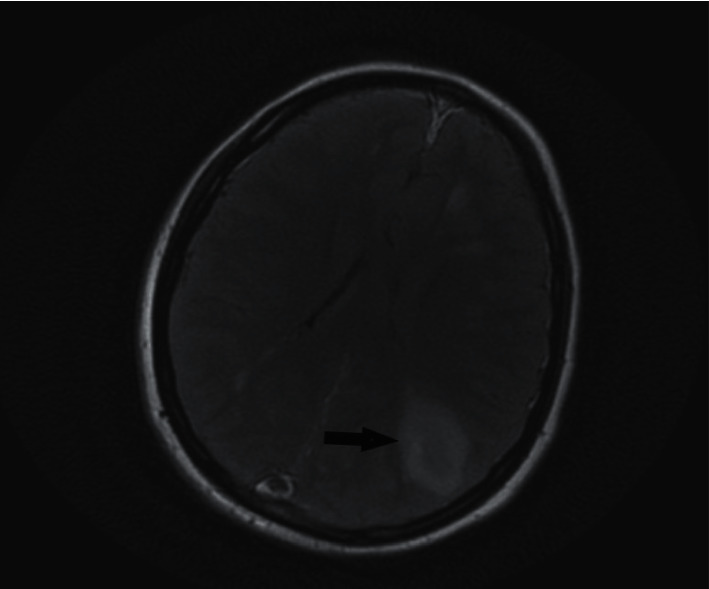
Magnetic resonance imaging (MRI) of the brain with contrast, T2 flair showing left parietal lobe hemorrhagic infarct (arrow).
